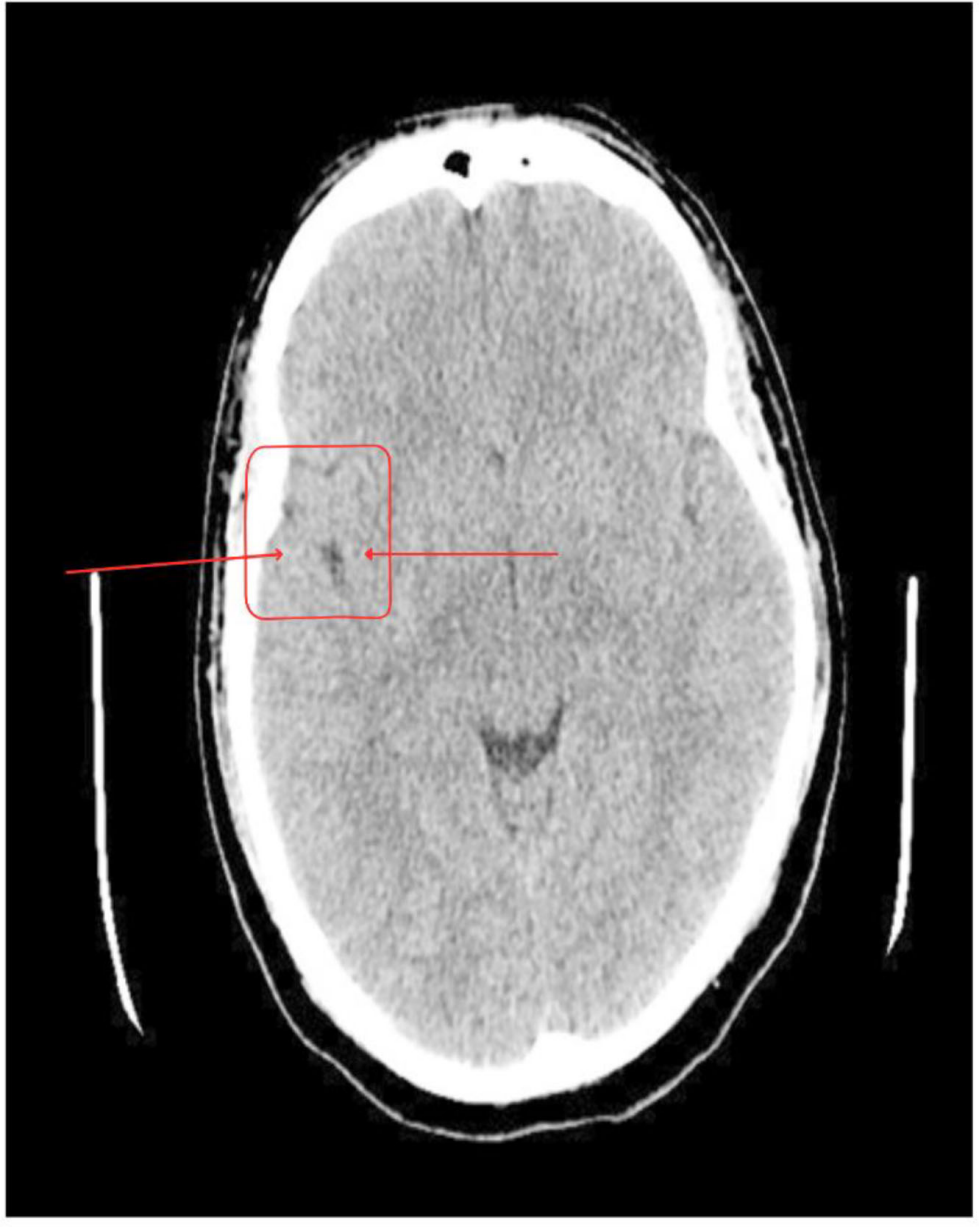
CT Brain (14 September 2025): A 3 mm cortical calcification with perilesional edema in the inferior posterior left frontal lobe, consistent with calcified neurocysticercosis.
